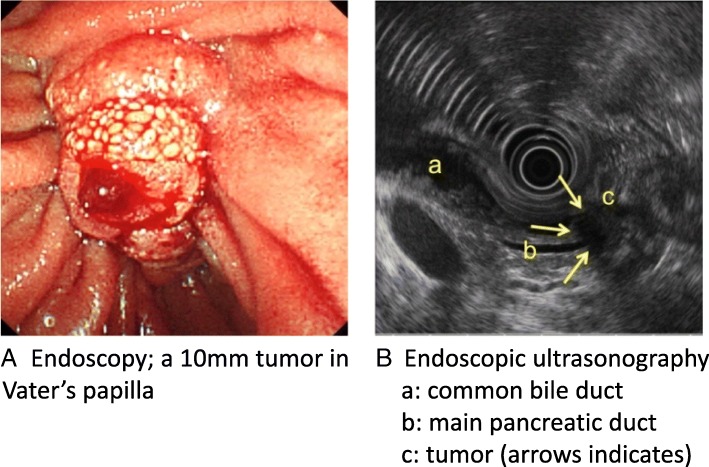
Preoperative endoscopy. Endoscopy revealed a 10-mm tumor in Vater’s papilla and endoscopic retrograde biliary drainage was placed (A). Endoscopic ultrasonography revealed that the tumor (arrows) invaded pancreatic parenchyma (B). a, common bile duct; b, main pancreatic duct; c, duodenum
